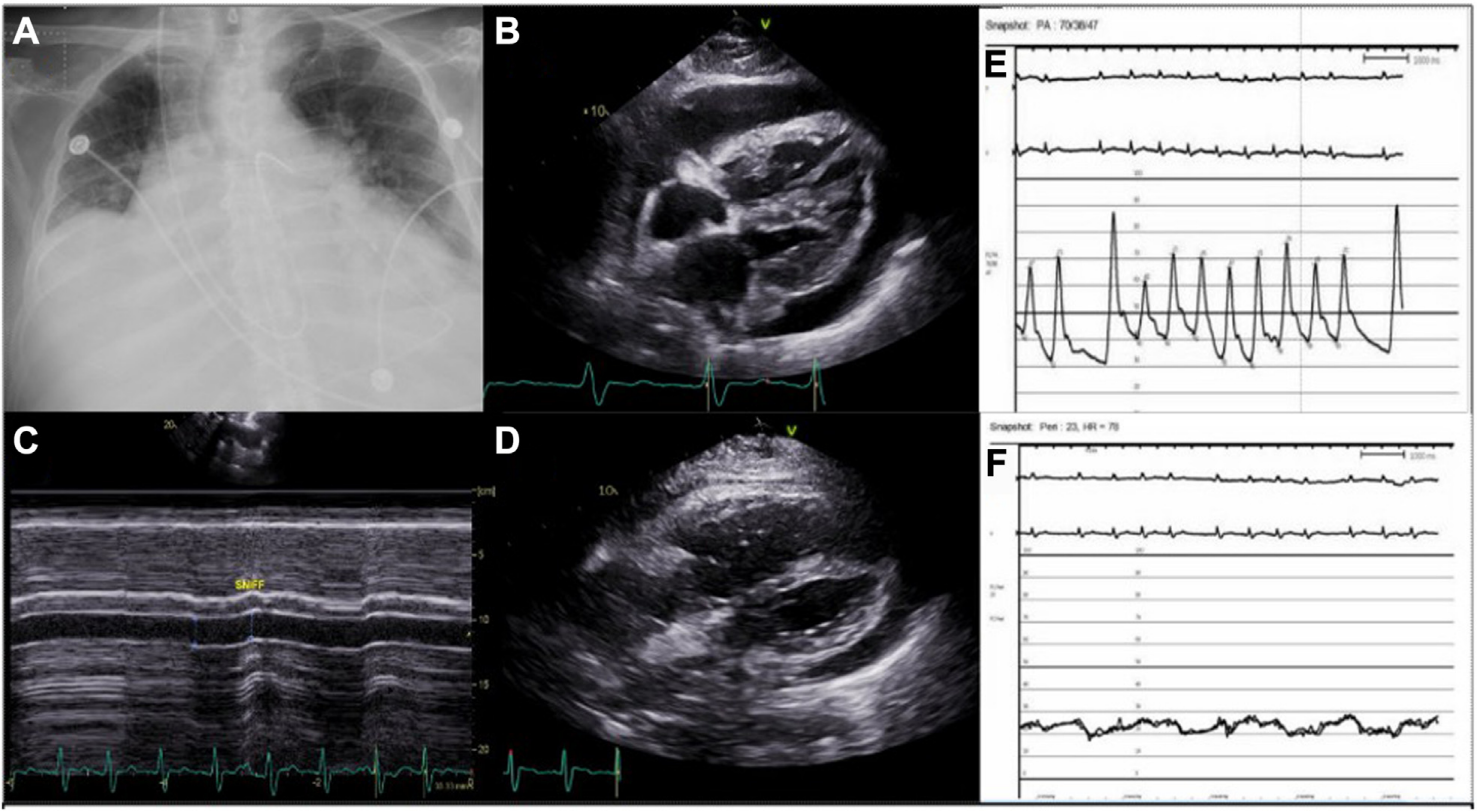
Management of cardiac tamponade in pulmonary hypertension. (A) Chest x-ray demonstrating bilateral pleural effusions with pulmonary edema and right heart catheter in situ. (B) Predrainage transthoracic echocardiogram (TTE) demonstrating large pericardial effusion with right ventricular (RV) collapse tamponade physiology (subcostal view). (C) Inferior vena cava of >21.0 mm without variation on sniff test. (D) Postconservative drainage TTE demonstrating interval decrease in the pericardial effusion with improvement on RV function. (E) Hemodynamic tracings initially with pulmonary artery pressure of 70/36 (47) mm Hg. (F) Opening pericardial pressure of 23 mm Hg.
